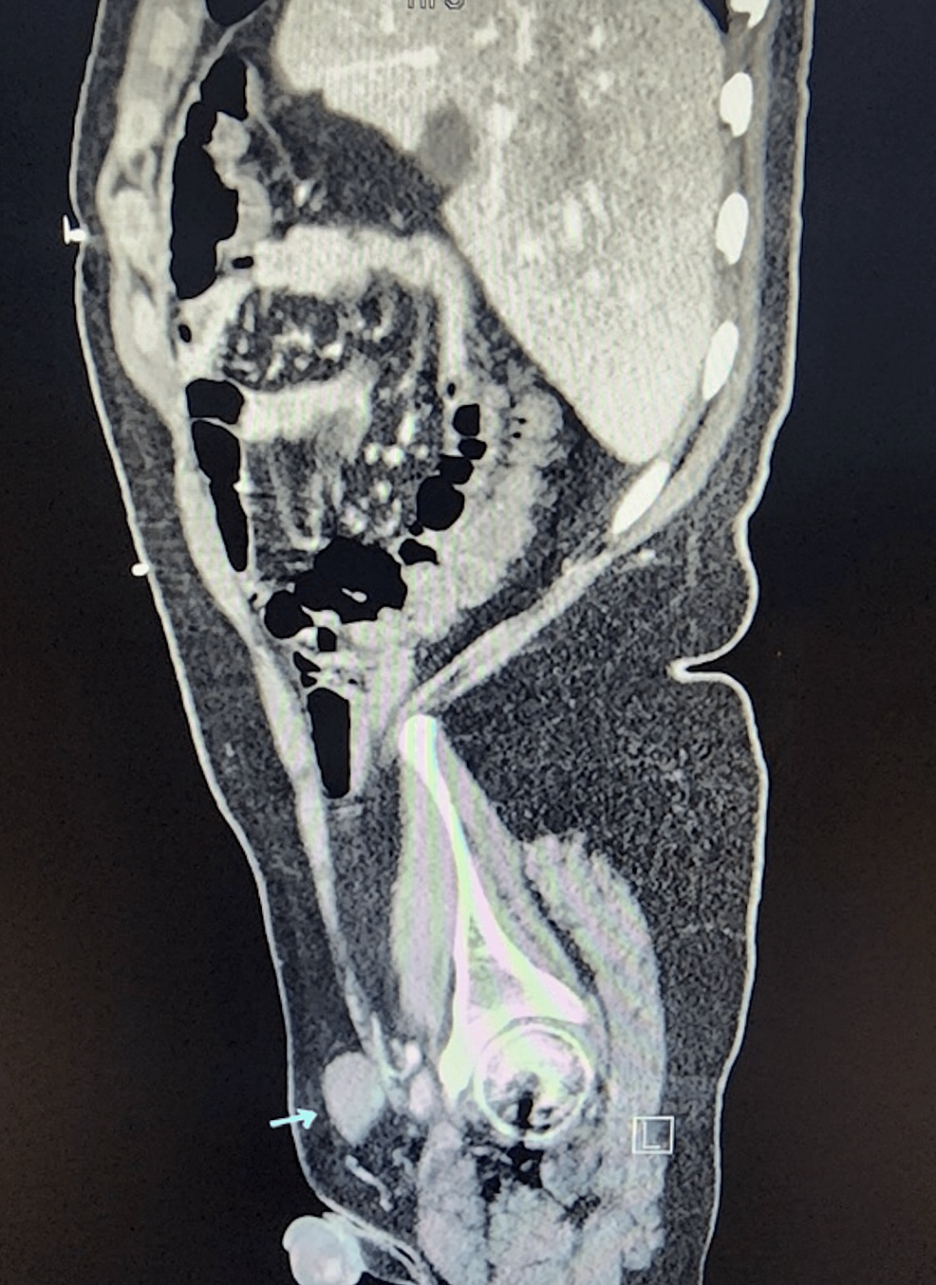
CT scan sagittal view. Blue arrow showing the undescended testicle.
